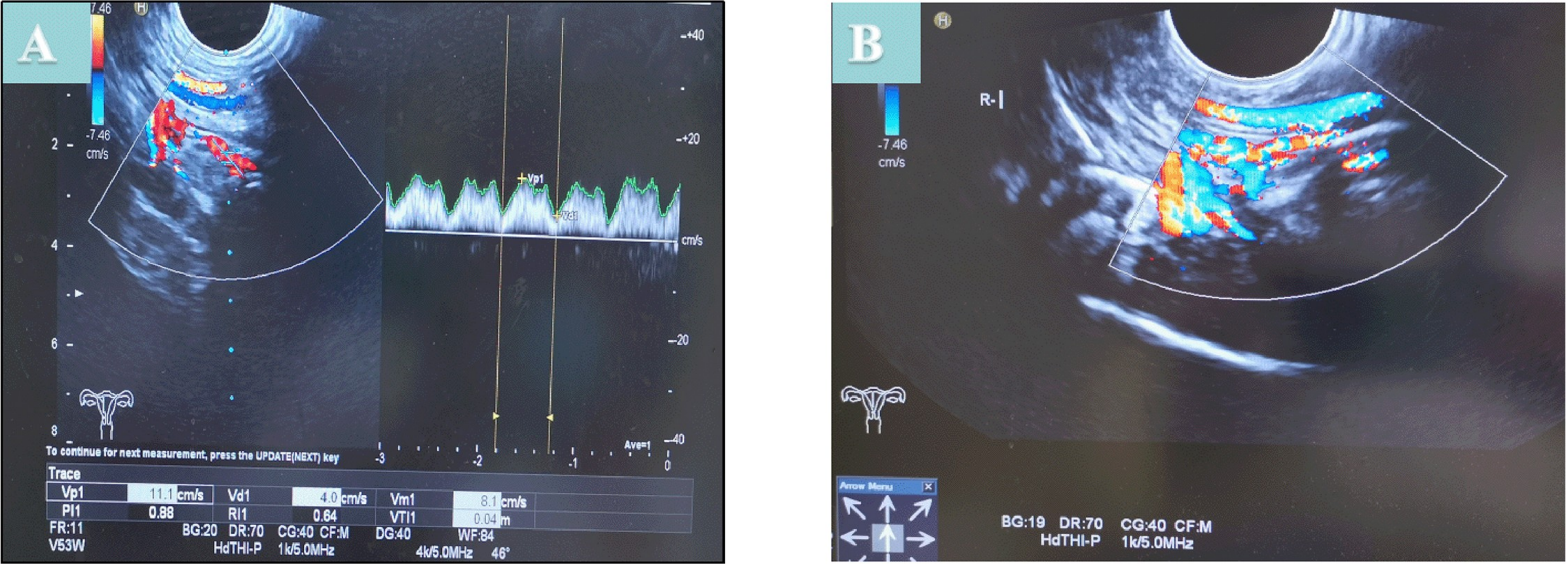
The blood flow of the transplanted uterus was evaluated by transabdominal ultrasonography.
